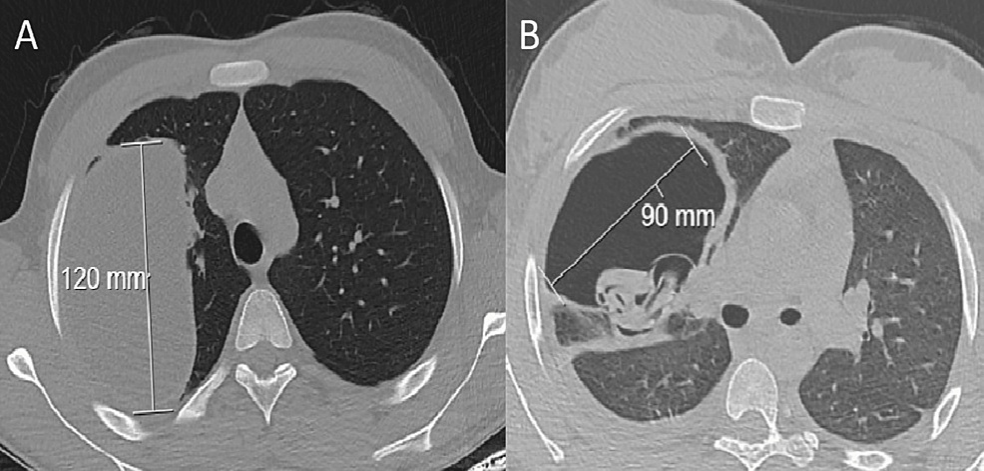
CT image of ruptured and non-ruptured pulmonary cysts (A) Axial chest CT image in the parenchymal window showing an uncomplicated hydatid cyst of the right lung. (B) Axial chest CT image in the parenchymal window showing a ruptured hydatid cyst of the right lung. Air in the cyst caused by bronchial rupture of the cyst and germinative membrane (water lily sign) can be seen.
